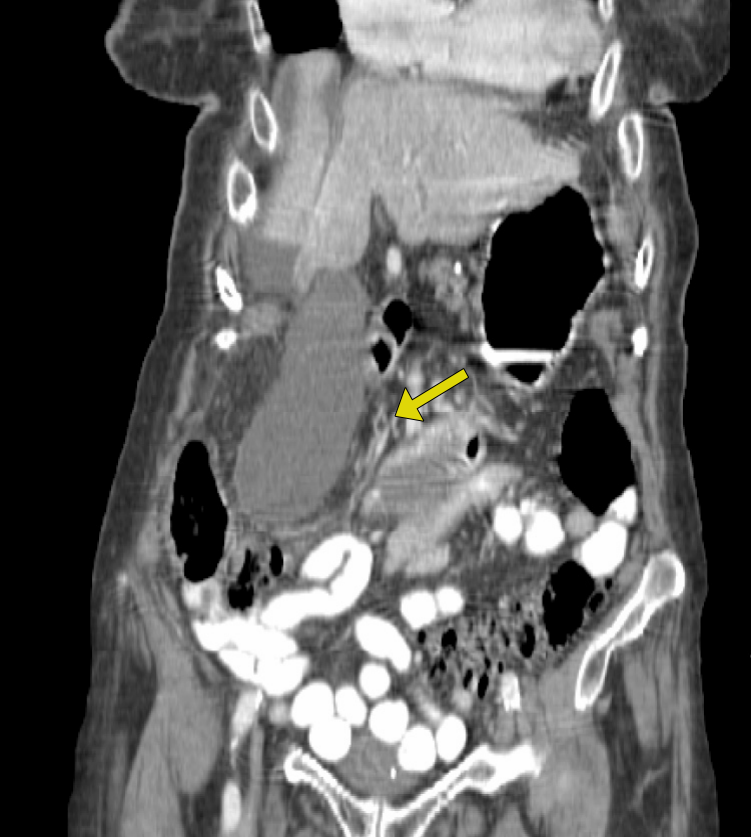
Computed tomography scan in sagittal section demonstrating a large hydropic gallbladder.
